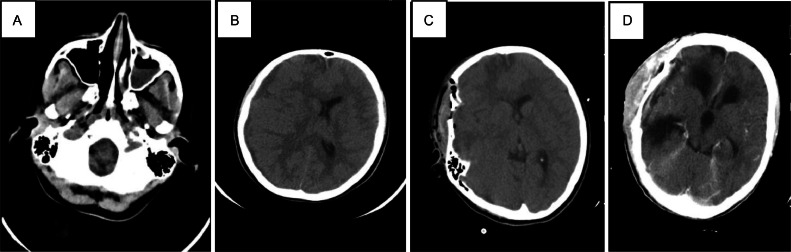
Brain CT scan without contrast (A) air-fluid level in left maxillary sinus suggestive of sinusitis (B) preoperatively showed A hypoattenuating extra-axial fluid collection with a diameter of 7.5 mm over the right hemisphere with a compressive effect on the lateral ventricle and midline shift of 7 mm into the left side was present, considered as subdural empyema. (C) Early postoperative CT scan showed reduced midline shift and drainage of a subdural extra-axial fluid collection, which was diagnosed as subdural empyema. Contrast-enhanced brain CT scan (D) 6 months after surgery showed noncommunicating hydrocephalus.
